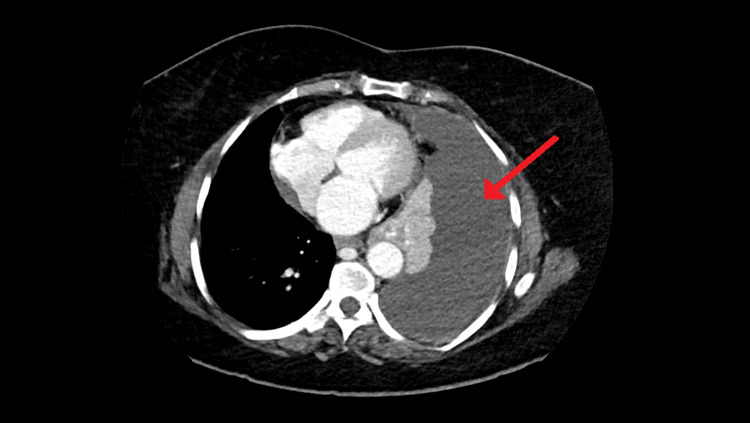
Large left pleural effusion seen on chest computed tomography
